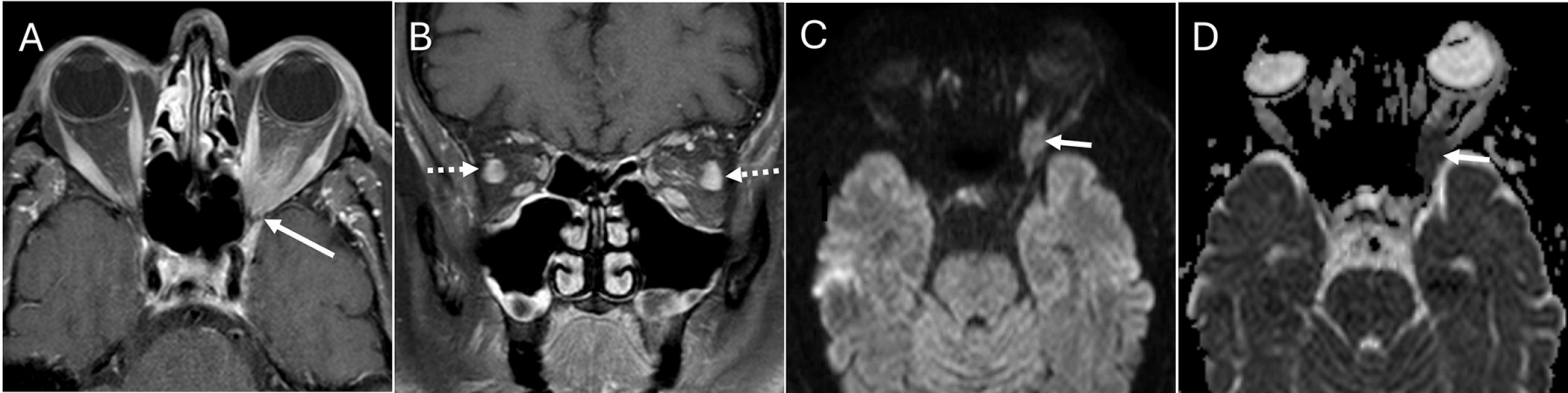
Orbital inflammation. Axial (A) and coronal (B) T1 post-contrast fat saturated images in a 72 year-old male show a left orbital apex mass extending intracranially (white arrows, A,C,D). Mild inflammatory stranding in the pre- & post-septal orbit and mild lateral rectus muscle enlargement (dashed white arrows, B) led one radiologist to conclude that the orbital apex mass was inflammatory, while the other radiologist felt the mass-like appearance favored neoplasm. Diffusion-weighted imaging (DWI) (C) and apparent diffusion coefficient (ADC) (D) show intermediate signal close to brain(white arrows). The confirmed clinical diagnosis was non-specific orbital inflammation.
